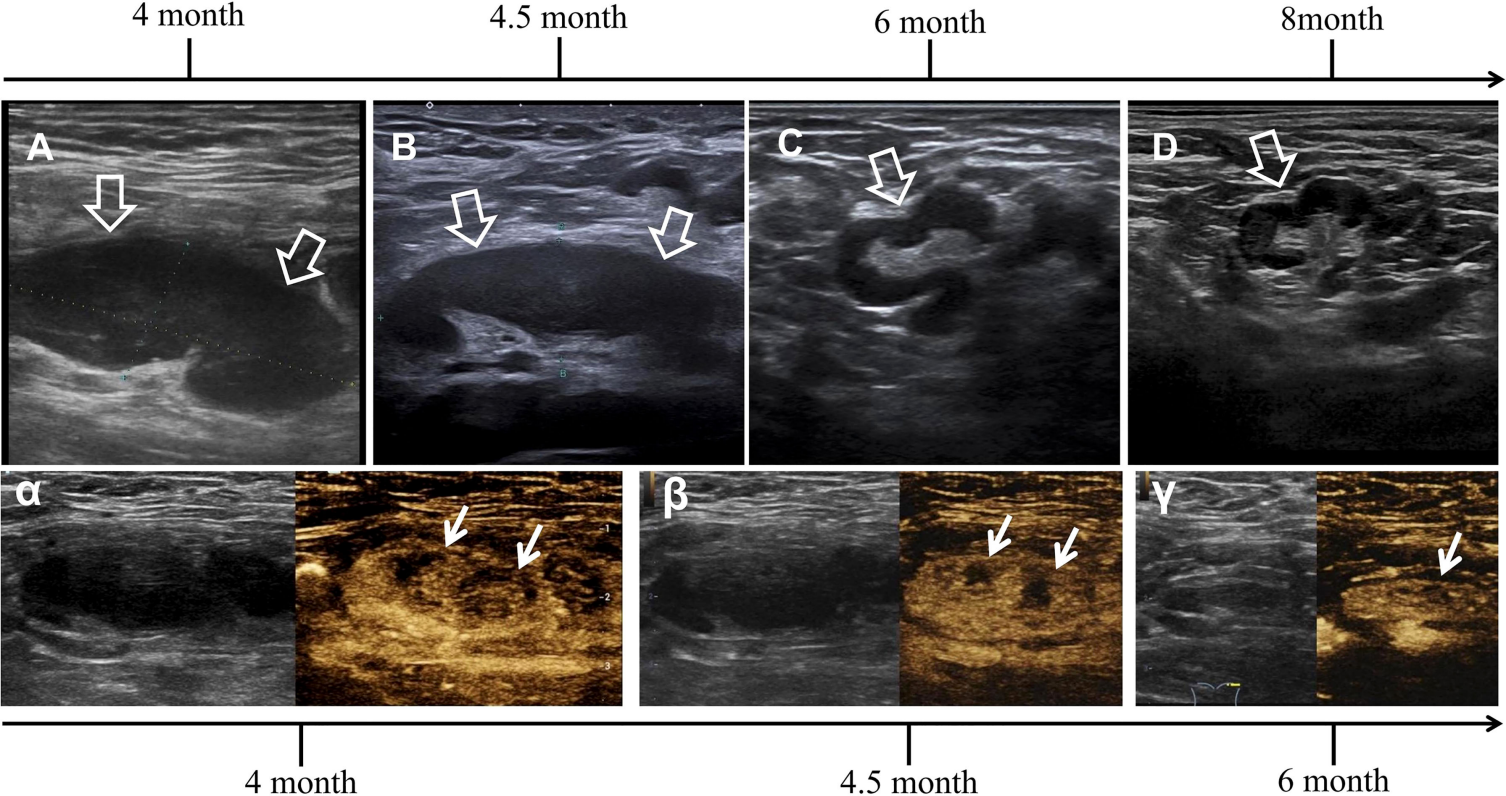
Successive ultrasonography (US) and contrast-enhanced ultrasonography (CEUS) images of the same largest lymph node, with dynamic changes during progression and regression. (A) The initial US showed the lymph node was in deep position, flat oval in shape, 40 mm on the long axis and 15 mm on the short axis, with a long/short ratio of >2. The lymphatic cortex was notably thickened to 12 mm and presented as a homogeneous hypoechoic area with a visible lymphatic hilum. (B) The second US showed indistinctive decrease in cortical thickness and the same lymph node size from the previous examination. (C) The third US in the follow-up showed a distinctive decrease in lymph node size and cortical thickness having irregular shape. (D) The last US showed normalized lymph node (indicated by hollow arrows). (α) The initial diagnosis of CEUS was based on centripetal perfusion enhancement in the asynchronous type, with a notable area in the deviated center showing hypoperfusion, covering half of the area of the lymph node. (β) The second CEUS showed distinctive decrease in hypoperfusion area. (γ) The third CEUS in the follow-up showed normalized enhancement and near invisibility of the hypoperfusion area (indicated by arrows).
